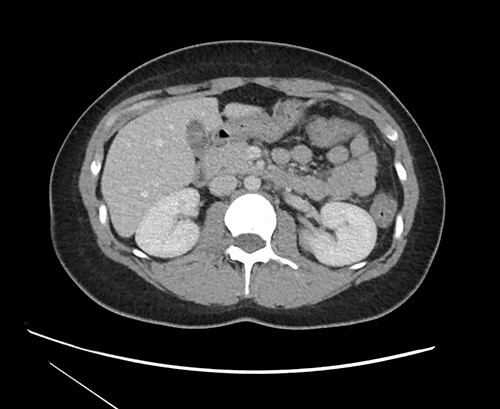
CT imaging at time of presentation to the ED showing multiple septations within the gallbladder.
